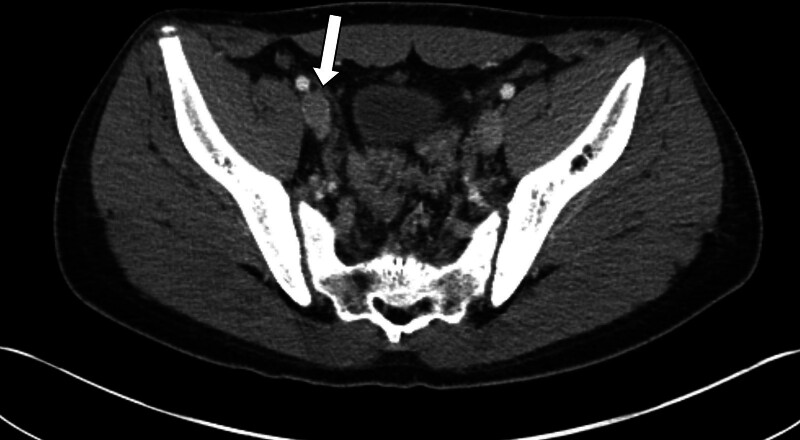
CT image of pre-anticoagulation. Thrombus at right external iliac vein. CT = computed tomography.
